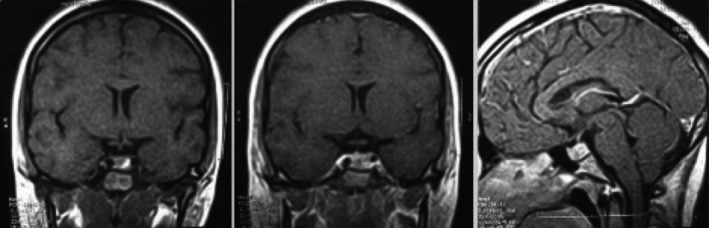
Coronal and sagittal T1 weighted MRI image 3 months after bromocriptine treatment shows a decrease in tumor size.
